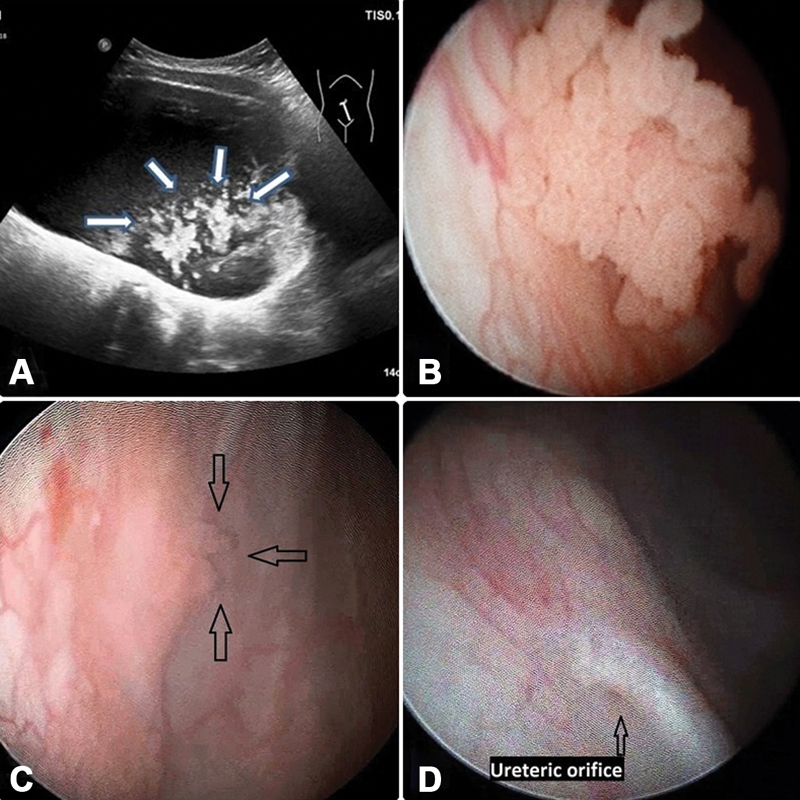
Next to the orifice of the right ureter, an 18 × 20 × 21 mm large, lobulated, and hyperreflective lesion was detected on ultrasound. (
